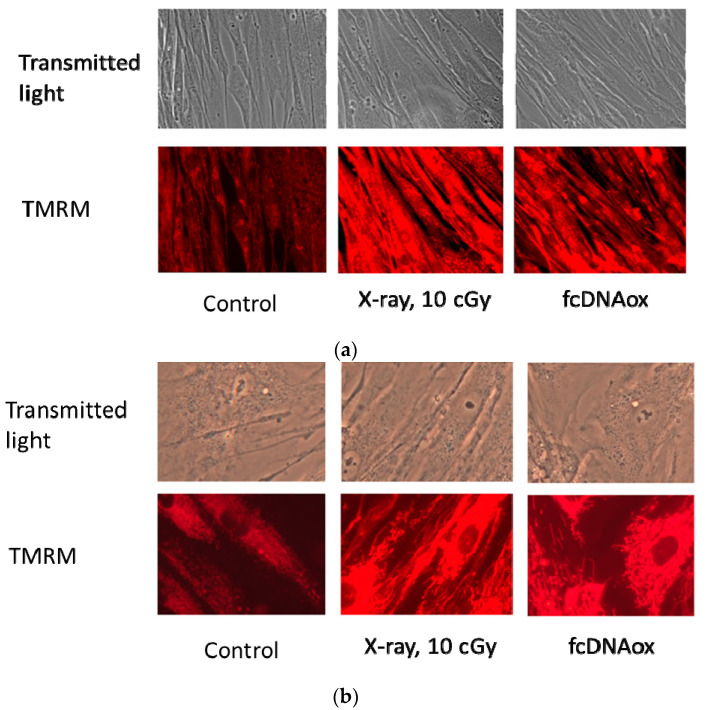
Mitochondrial potential in MSCs one hour after exposure to X-ray radiation (10 cGy) or oxidized cfDNA (50 ng/mL); (a) the magnification is 40×, (b) the magnification is 100×.
